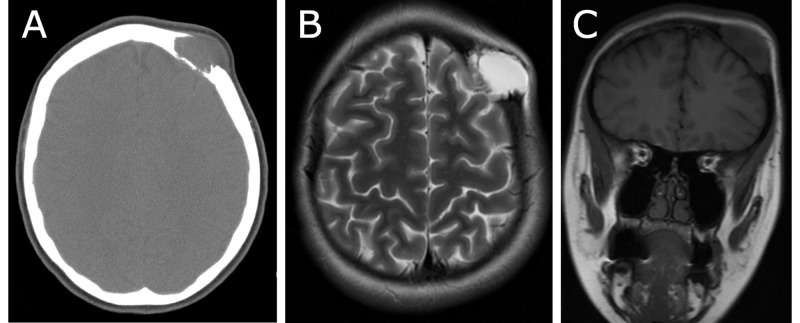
CT scan and MRI prior to surgery. A: Axial CT scan of the head without contrast, B: Axial T2-weighted MRI scan of the head, C: Coronal T1-weighted MRI scan of the head. CT: computed tomography; MRI: magnetic resonance imaging
